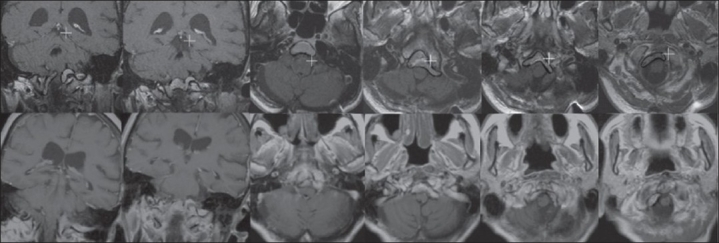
Row 1: At the time of the gamma knife radiosurgery (GKRS), the patient had a meningioma with a volume of 6.8 cc extending en plaque from the junction of the medulla and the pons into the foramen magnum along the clivus. Row 2: On follow-up imaging 13 years after GKRS, the lesion decreased in size to 4.0 cc without any new growth or radiographic complications of GKRS (cyst formation, necrosis, and edema)
